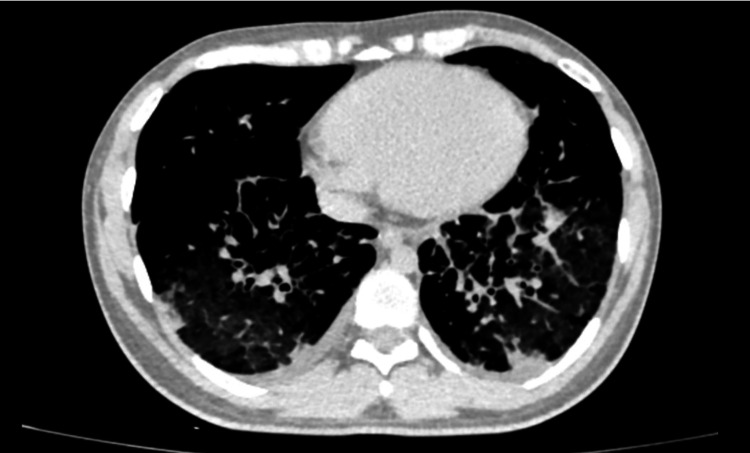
Chest computed tomography scan Consolidation in the right upper lobe with air bronchogram and posterior middle lobe with air bronchogram inside. In the left lung, the presence of consolidation with air bronchogram in the posterior region and in the posterior inferior lobe
